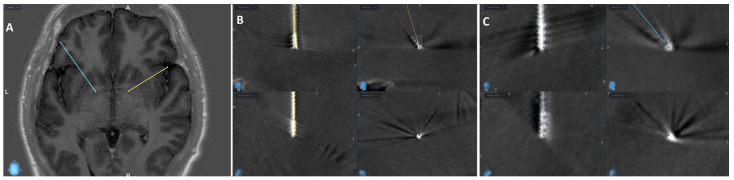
(A) Inverse recovery axial MR image of the planned targets for the second patient. (B,C) show the CT image after final lead placement for right (yellow) and left (blue) electrode, respectively, merged with the preoperative plan to confirm the final position. It is displayed in four different views: top left shows Trajectory 1, bottom left shows Trajectory 2, top right is the sagittal view, and bottom right is the probe’s eye view.
